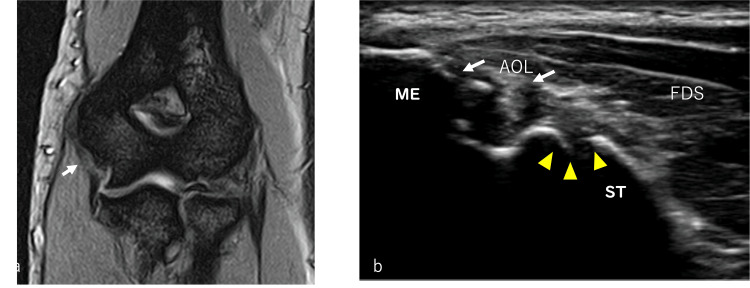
Magnetic Resonance Imaging (MRI) and ultrasound images of the injured site (a) MRI; (b) Ultrasound. On the affected side, an avulsion of the medial epicondyle and injury to the ulnar collateral ligament (UCL) can be observed (arrows). Yellow arrowhead: joint space. ME: medial epicondyle; ST: medial epicondyle to the sublime tubercle; FDS: flexor digitorum superficialis; AOL: anterior oblique ligament
